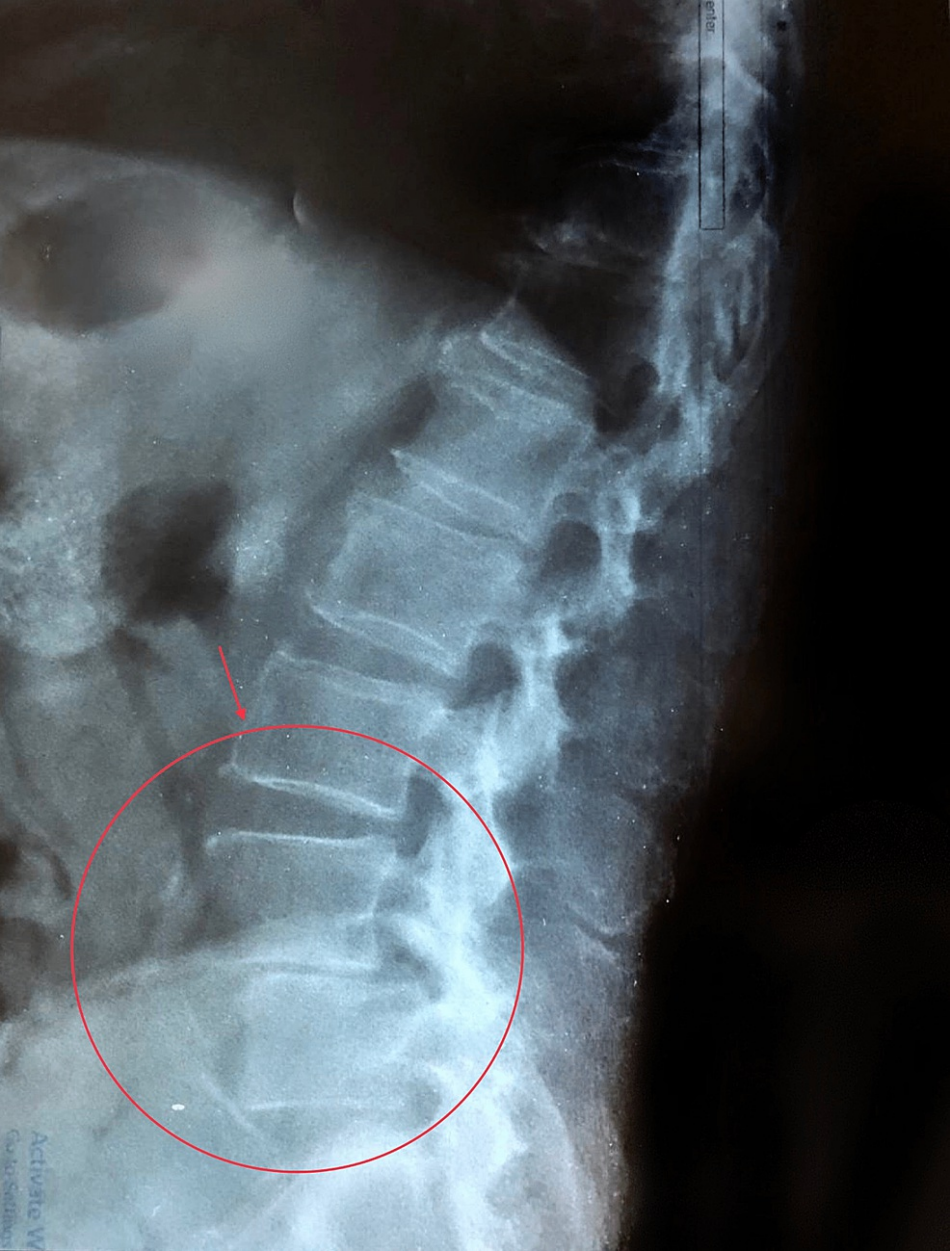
Plain lumbo-sacral radiograph showing L4-5 spondylolithesis and signs of osteoarthropathy.
